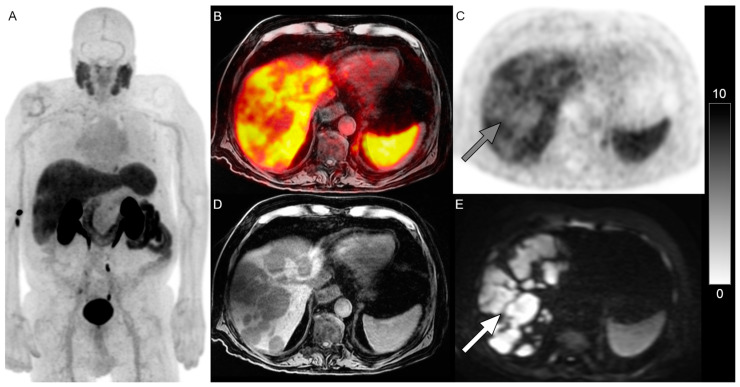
PET/MRI in a 78-year-old man with mCRPC with disease progression on cabazitaxel. Whole-body ^18^F-DCFPyL PSMA PET maximum-intensity projection image (A) shown. Extensive PSMA-negative hepatic metastases ((B), fused image, (C), gray arrow, PSMA PET) are identified on T1-weighted image (D) and b = 800 DWI ((E), white arrow). The scale bar of PET activity (SUVs) is on the right. Given the extent of PSMA-negative disease, RLT was not considered suitable. He died two months later.
